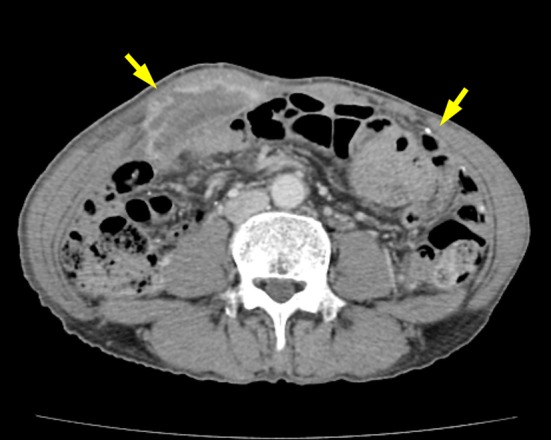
Abdominal computed tomographic scan after disease recurrence showing rim-enhanced lesions with calcification over rectus abdominis muscle and peritoneum (arrows).
